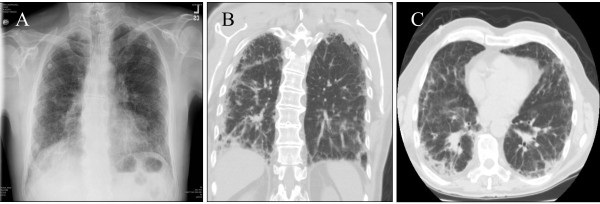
A. Plain chest radiograph shows bibasilar infiltrates with a peripheral reticulonodular pattern superimposed on generalized interstitial changes involving the upper lobes and lung bases. B. (Coronal view) and C. (Axial View) High Resolution Computed Tomography of the chest shows thickening of intralobular septa, septal line formation, parenchymal band formation and peribronchial thickening. Mild mediastinal lymphadenopathy is also noted.
